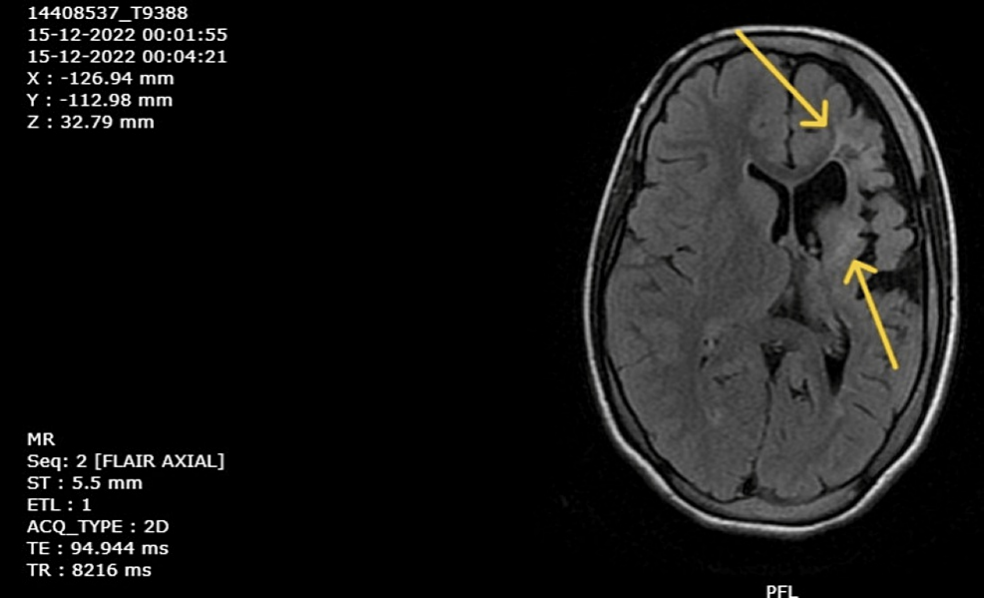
MRI scan of brain showing gliotic areas (December 10, 2022).
